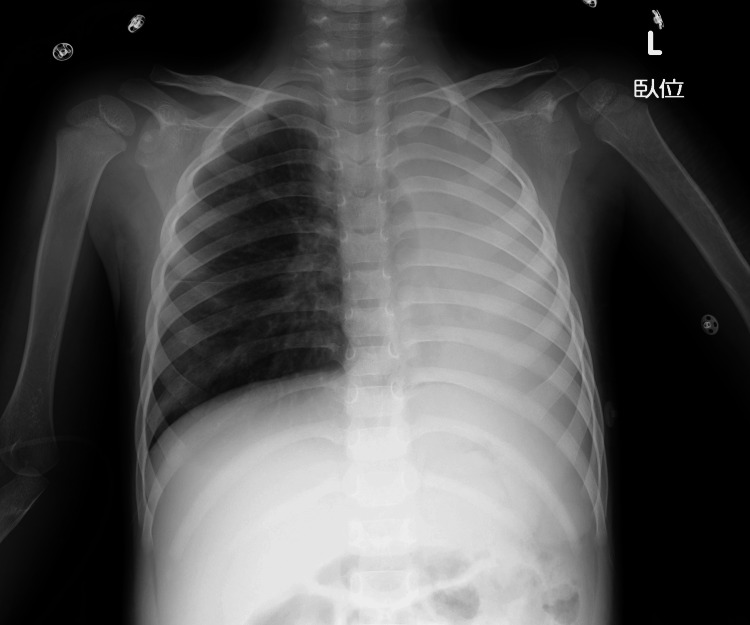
Pre-intubation chest X-ray Admission image showing total atelectasis of the left lung
